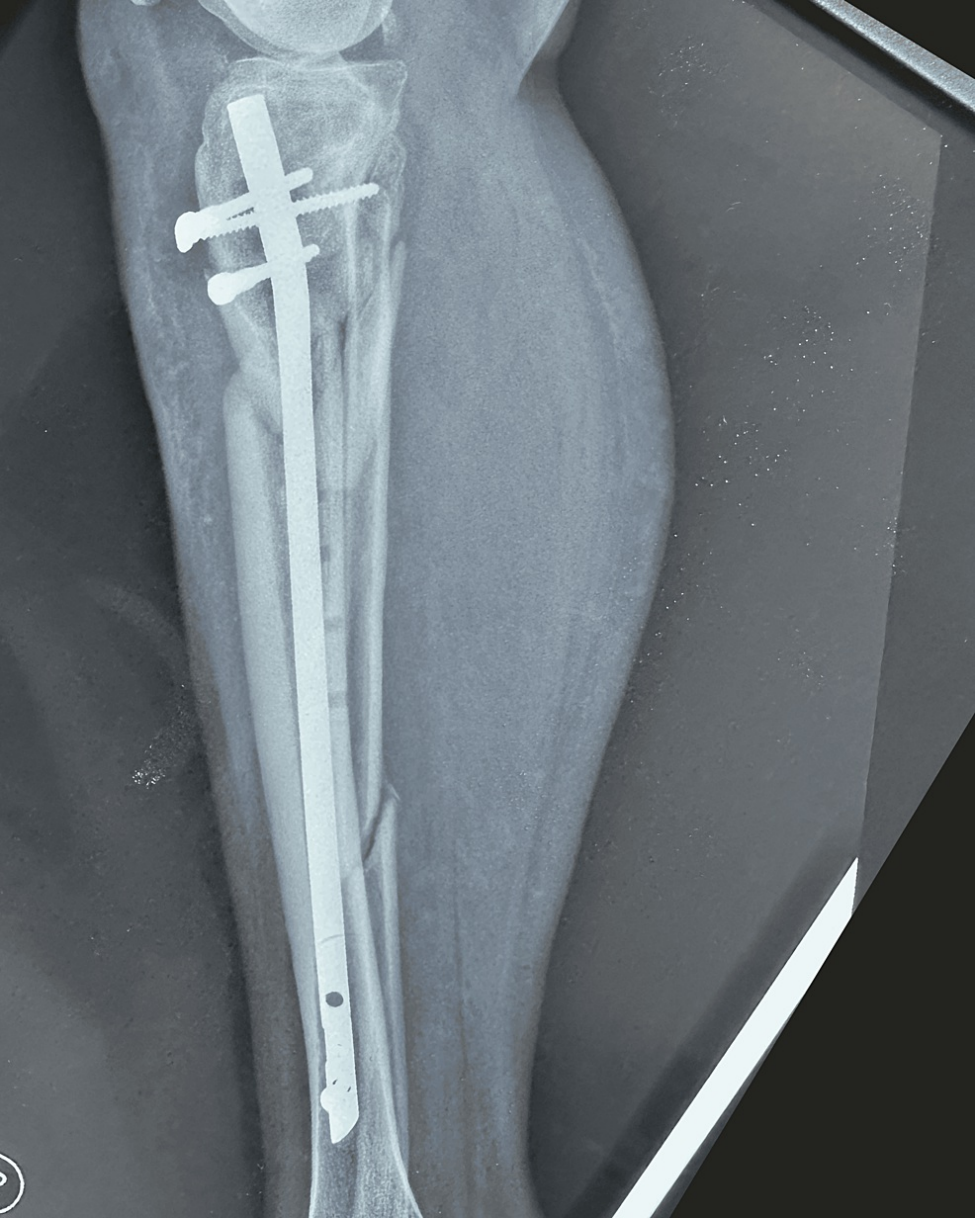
One-month postoperative lateral X-ray of the right leg showing the antegrade tibial nail
